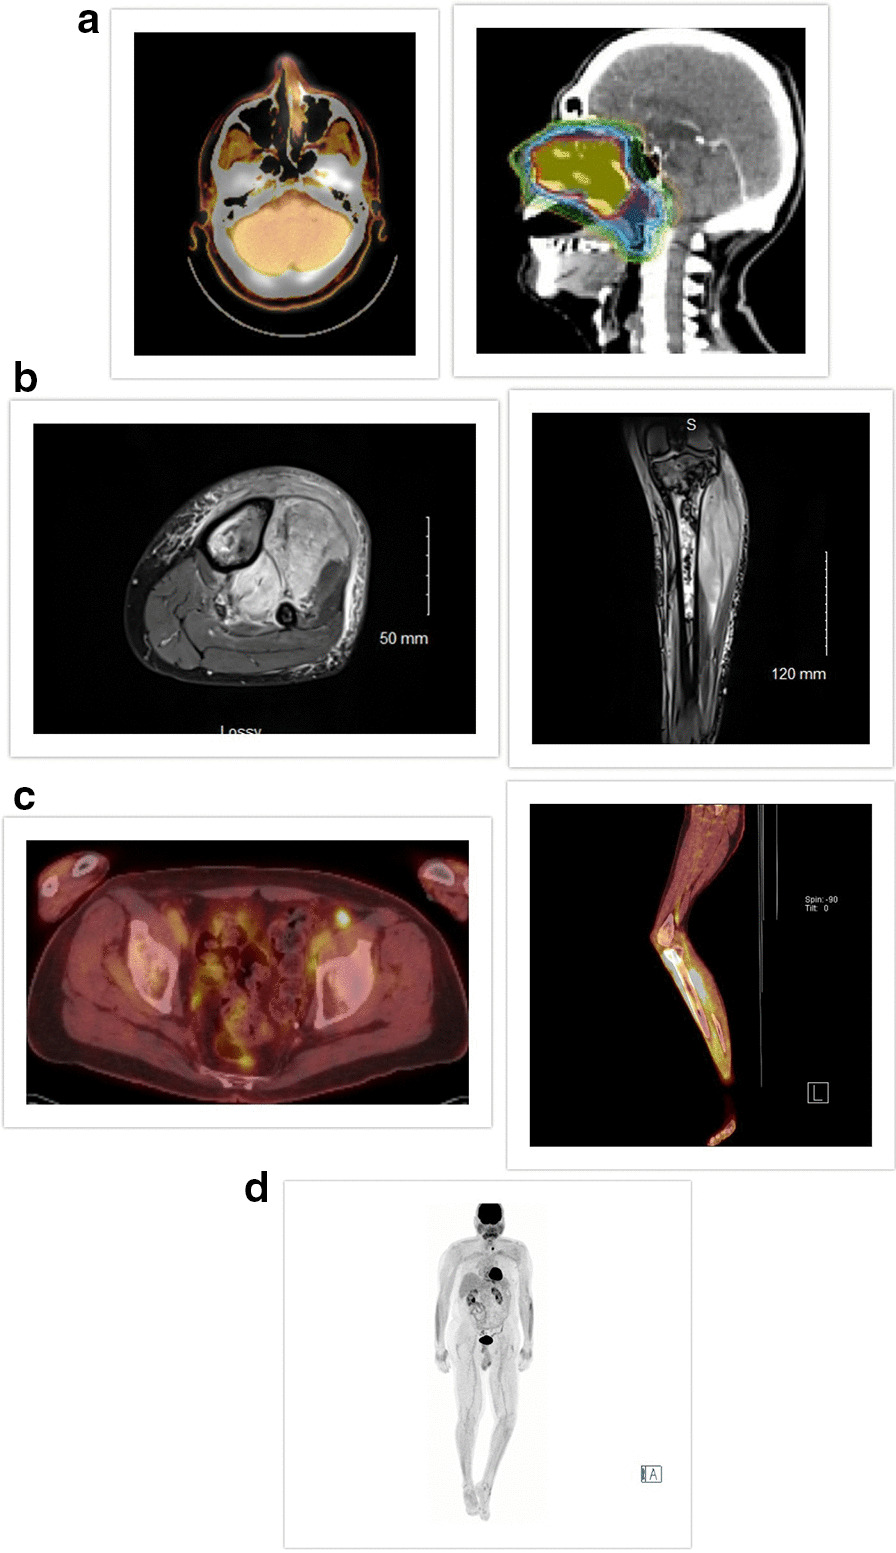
a Imaging showing “limited” stage I ENKL involving left nare/maxillary sinus at diagnosis and IMRT field used for therapy in 2013. b Magnetic Resonance Imaging of left lower extremity at relapse in 2018 showing cortical erosion of the left proximal tibia with marrow signal abnormality extending from the knee joint to the mid tibial diaphysis and prominent soft tissue abnormality of the musculature. c Positron Emission Tomography-Computed Tomography showing the left proximal pretibial mass measuring 9.4 × 9.7 cm (SUVmax 9.6) with FDG avid lymph nodes in the left inguinal and external iliac regions (SUVmax 4.82). d End-of-treatment Positron Emission Tomography- Computed Tomography showing evidence of complete metabolic remission
